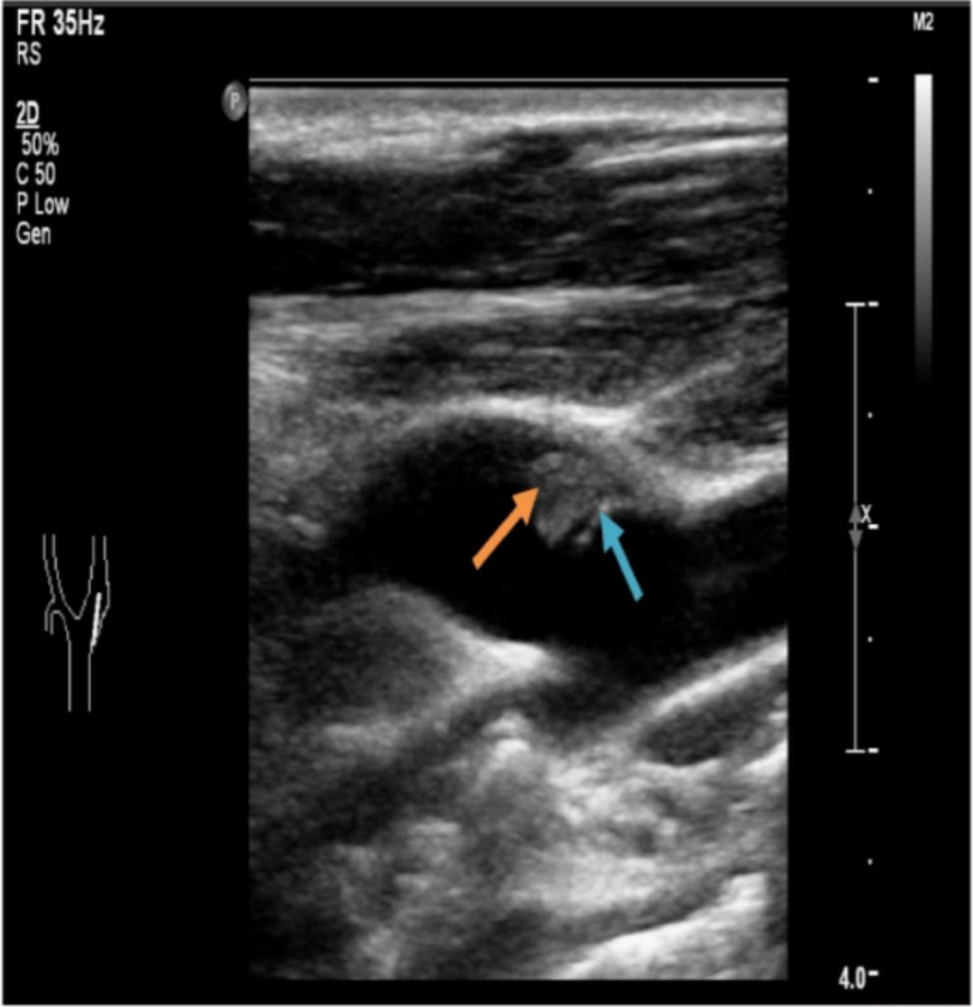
Carotid ultrasound showed CaW (blue arrow) and a new thrombus (orange arrow)
